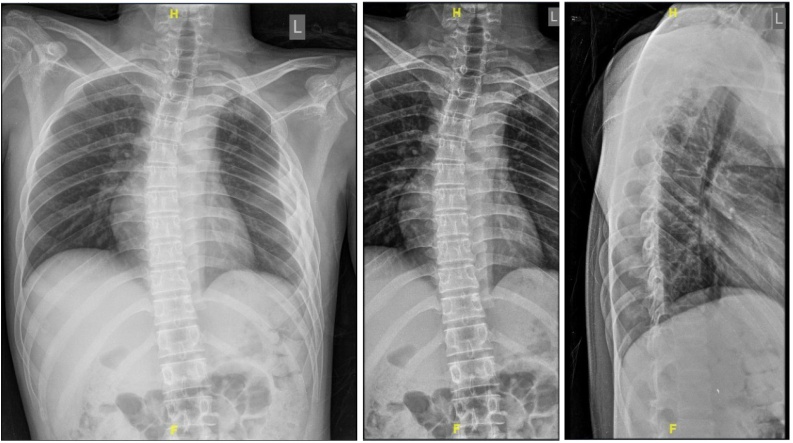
Plain X-Ray. Left: Chest X-Ray AP view showed homogenous consolidation at the right upper lung. Middle: Thoracolumbal X-Ray AP view showed normal finding. Right: Thoracic X-Ray lateral view demonstrated no abnormalities.
